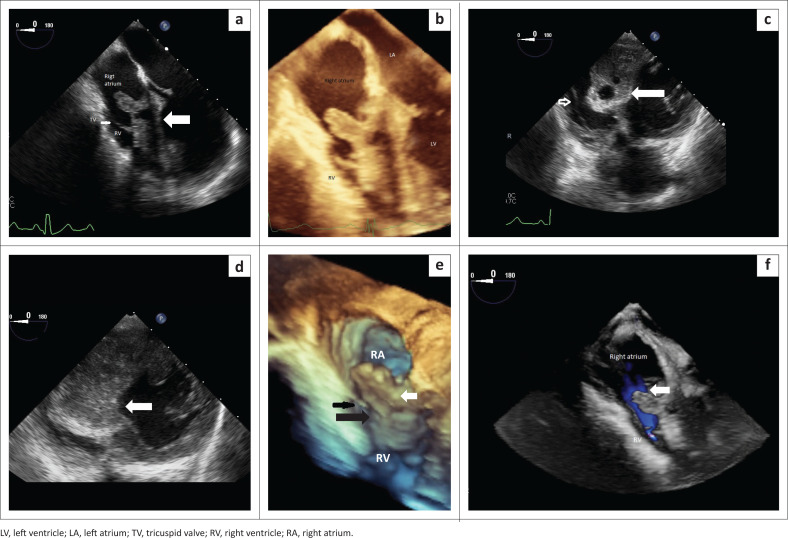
(a) Two- and three-dimensional transoesophageal echocardiography view of the heart at the mid-oesophageal level showing infiltration of the entire interventricular septum (open white arrow) by the hydatid cyst and reduction of the right ventricle to a slit-like cavity. (b) The hydatid cyst protrudes into the right atrium without causing tricuspid valve dysfunction. (c and d) Two-dimensional transoesophageal echocardiography trans-gastric view showing infiltration of the interventricular septum by the hydatid cyst (solid white arrow) and its relation to the tricuspid valve (open arrow, c). Deep trans-gastric view depicting septal hypertrophy due to hydatid disease (white arrow) mimicking asymmetrical septal hypertrophic cardiomyopathy (d). (e and f) Three-dimensional echocardiographic reconstruction of the right atrium (RA) and right ventricle (RV) showing the anterior location of the hydatid cyst protruding into the right atrium (white arrow) relative to the tricuspid valve (black arrow) at the base of the heart (e), and unobstructed blood flow through the tricuspid valve (white arrow) (f).
